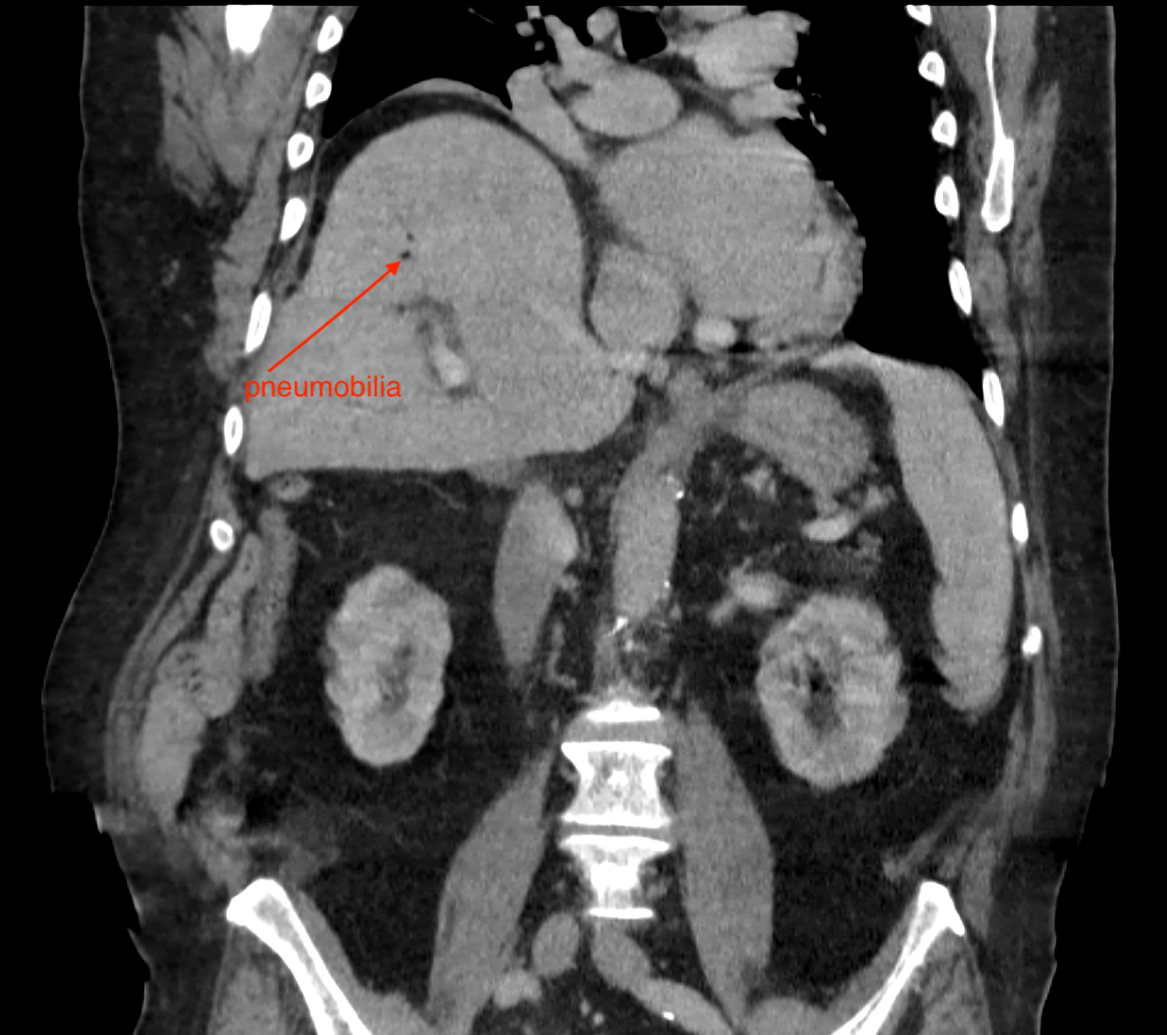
Coronal CT image showing pneumobilia
